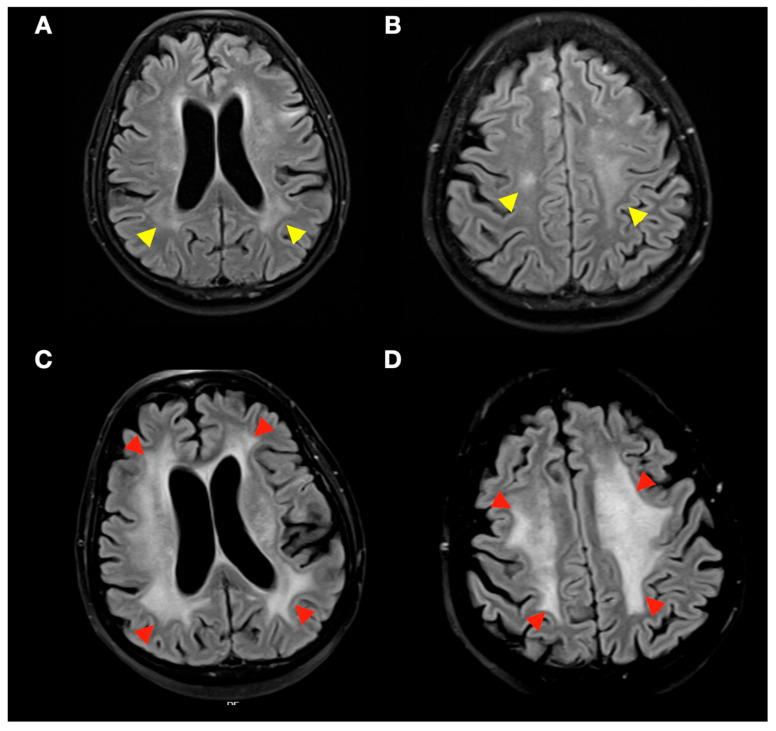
Treatment planning with VMAT-fSRS and treatment response. Axial FLAIR MRI. (A,B) (top): Yellow arrowheads indicate incipient signs of radiation-induced leukoencephalopathy after prior WBRT. (C,D) (bottom): Red arrowheads show progression after SRS in patients with synchronous infratentorial and supratentorial treatment. Predominant involvement of the white matter is seen in the corona radiata (A,C) and centrum semiovale (B,D).
